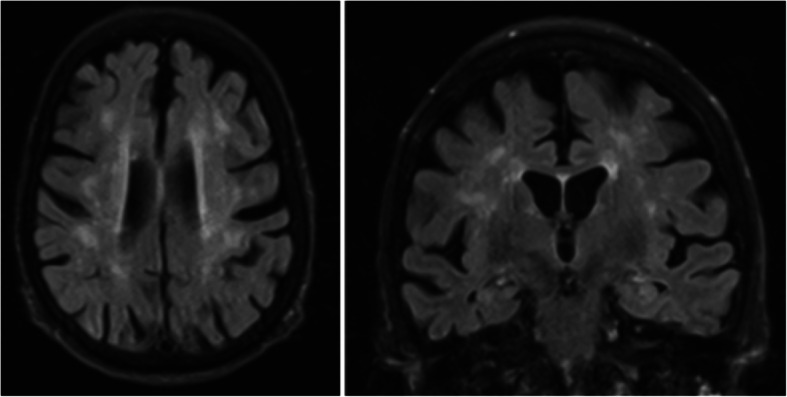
MRI brain. MRI without contrast demonstrates generalized atrophy and patchy periventricular and subcortical white matter hyperintensities
